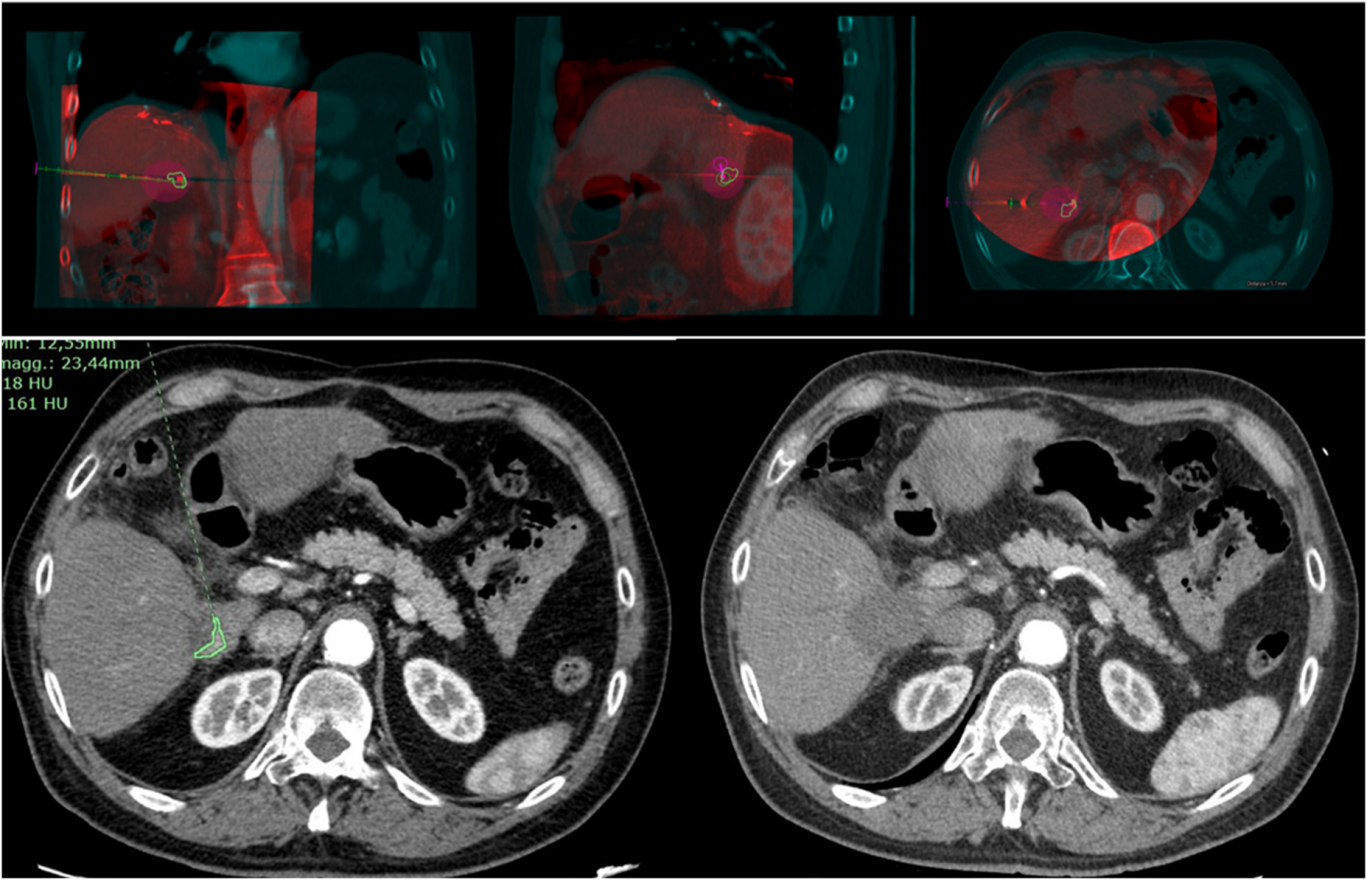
The first 3 images are CBCT + CT fusion (coronal, sagittal, axial) after antenna placement. The Volume Prediction (purple) shows adequate ablative margin. The images are 2 arterial axial CT scans: the first is before the procedure, with lesion segmentation (green) and the second CT is the FU, with complete ablation of the lesion as predicted by volume ablation software. Abbreviations: CBCT, cone beam computed tomography; CT, computed tomography; FU, follow up.
